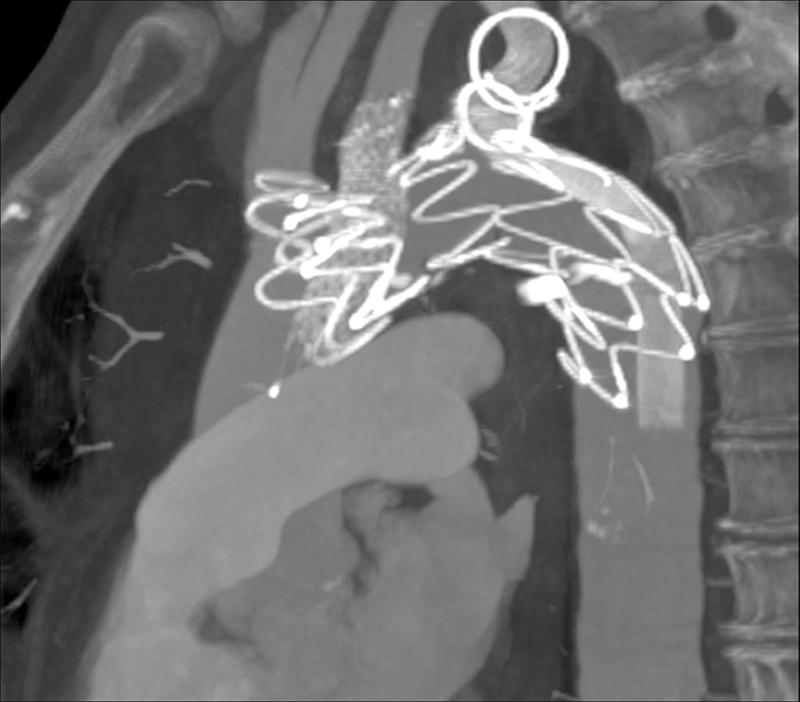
Three-year follow-up angio–computed tomography scan.
